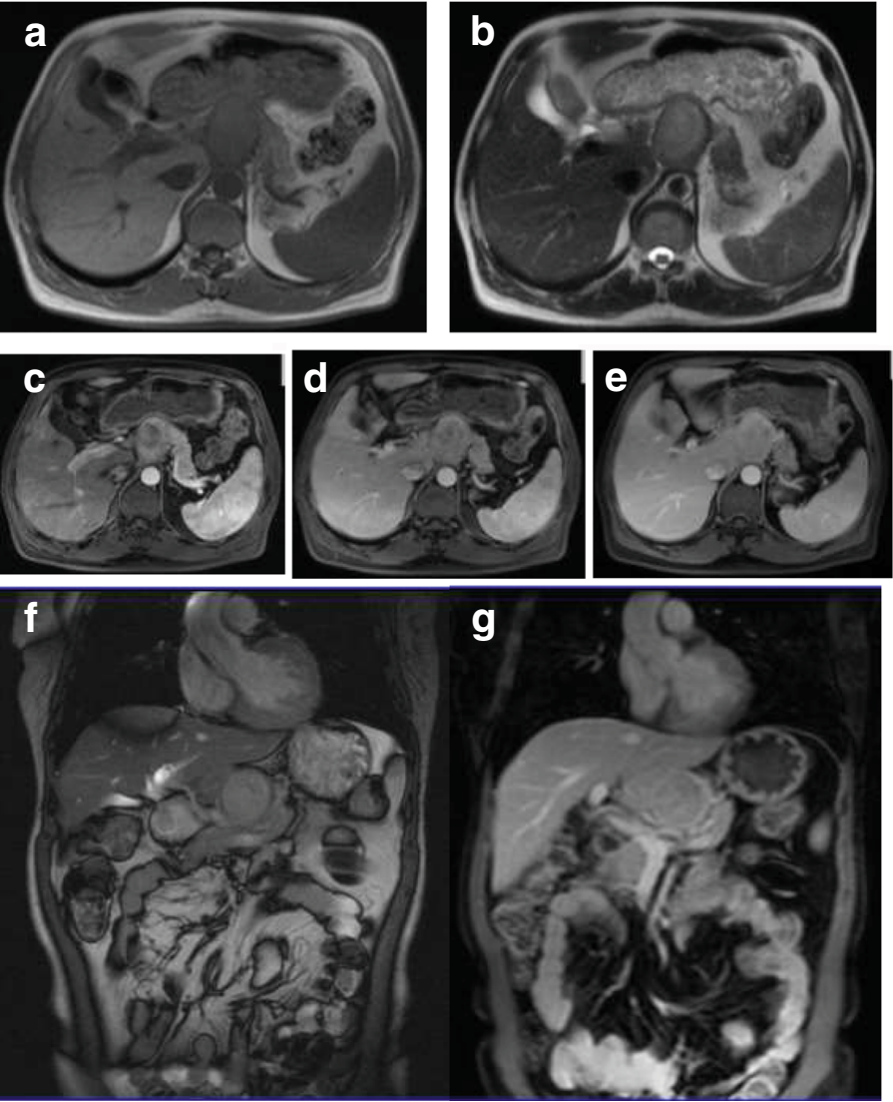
a-g MRI images showed a mass that was hypointense on T1WI and mildly hyperintense on T2WI in the pancreas with gradual, moderate, heterogeneous enhancement. The caudate lobe of the liver was compressed, and the mass had a poor margin with respect to the celiac trunk, abdominal aorta and the right crus of diaphragm
